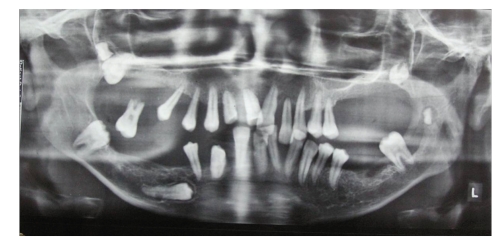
Panoramic radiograph showing generalized bone loss with floating teeth appearance.
